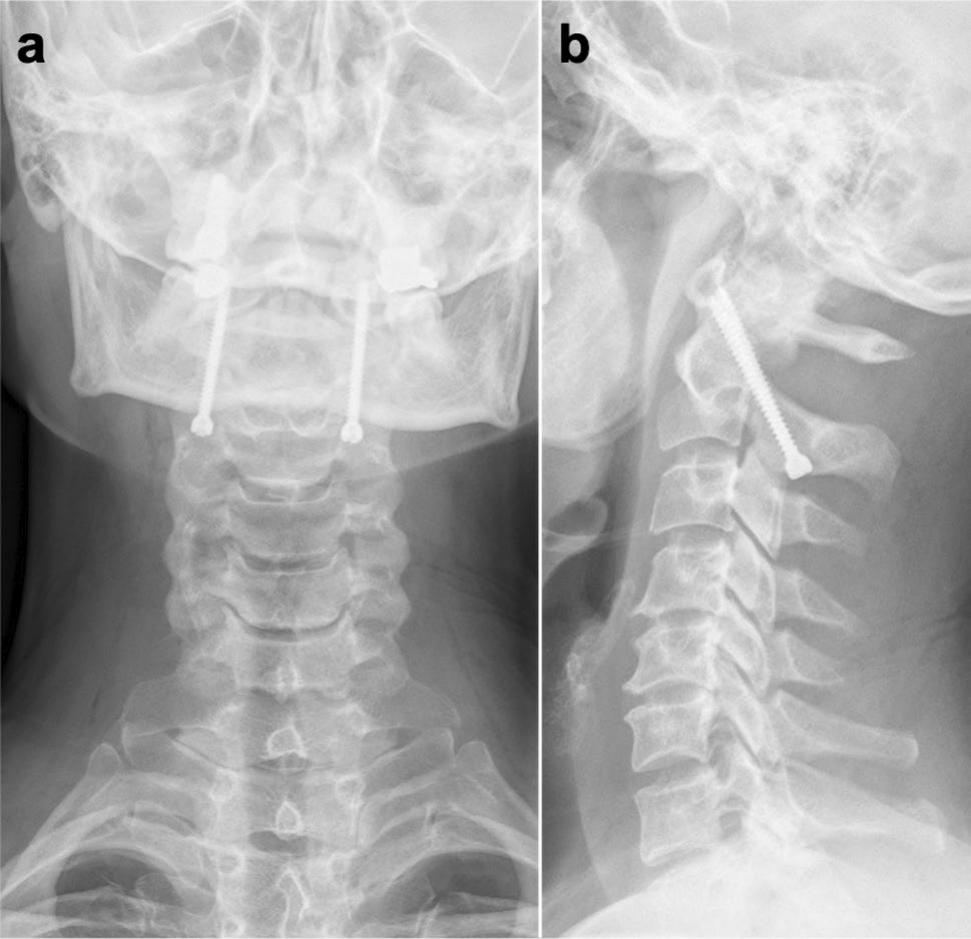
Correct screw position. This figure shows a typical example of a correct material position after isolated percutaneous transarticular screw fixation of C1 and C2 (IPTSFC1/C2). Conventional X-ray. (a) Anterioposterior plane. The screws inserted bridge the atlantoaxial joints on both sides and pass thrue the lateral mass of C1 and C2 on both sides. (b) Sagittal plane. The screws do not protrude more than 5 mm beyond the anterior arch of C1. If all these criteria match, the position of the screw is considered to be correct.
